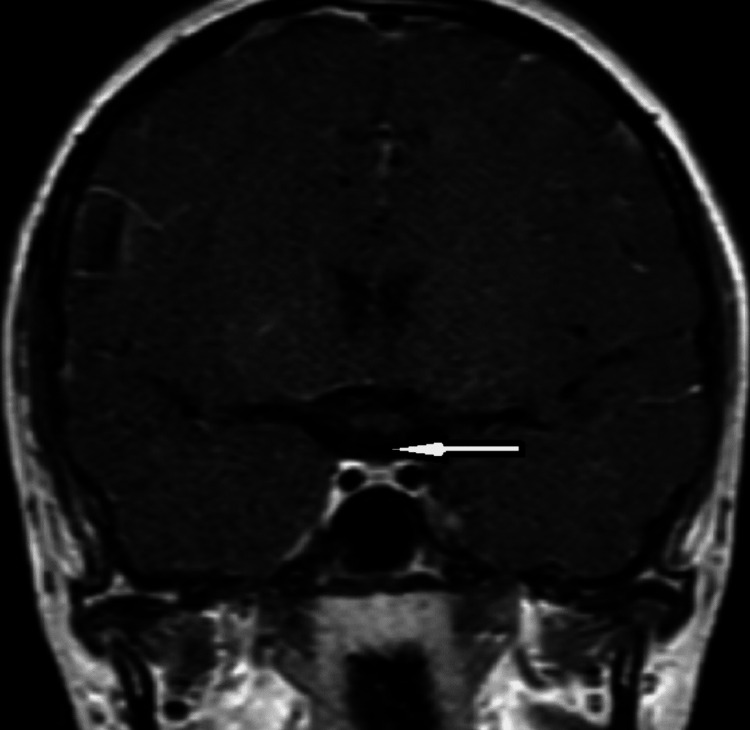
Coronal post-contrast magnetic resonance imaging of the brain shows that the pituitary stalk/infundibulum cannot be identified (as shown by the white arrow)
